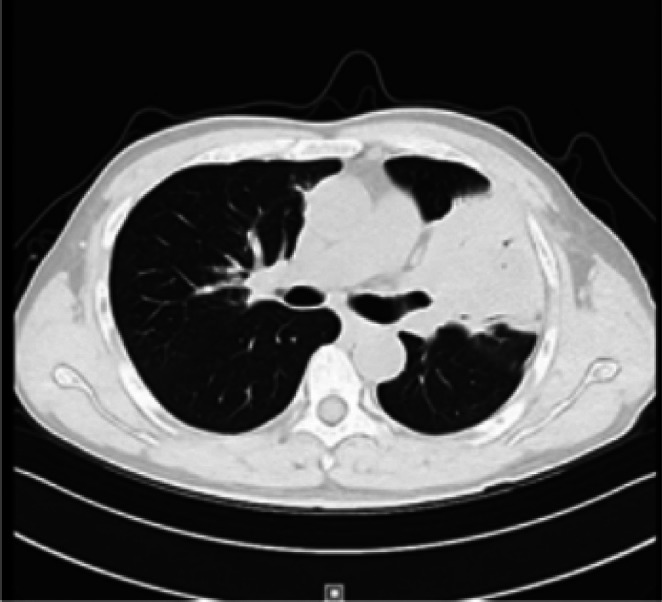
Chest CT suggests solid lesion in left upper lung.
